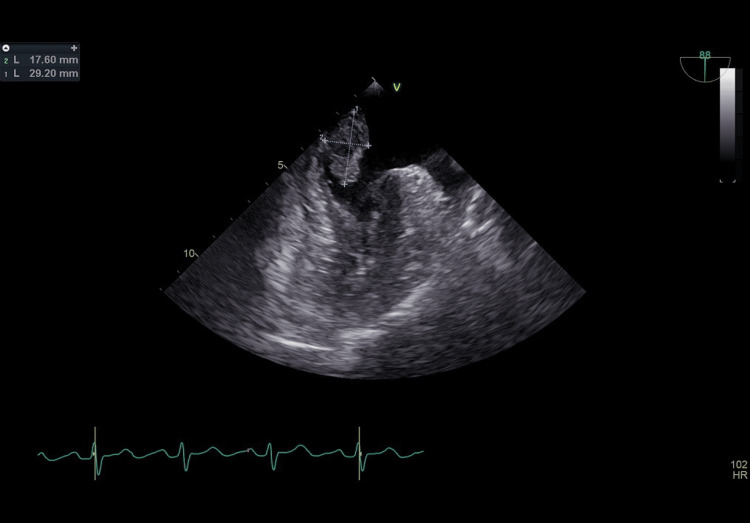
Transesophageal echo showing left atrial myxoma attached to the IAS of size 25*14mm (pedunculated) IAS: interatrial septum.
